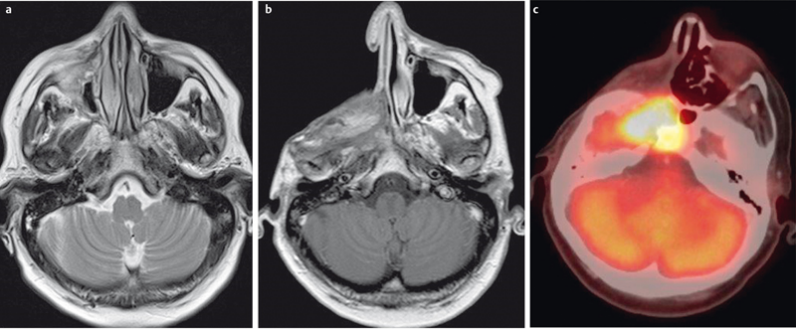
46-year-old female patient with chondrosarcoma of the right maxillary sinus. a) Preoperative axial T2w image demonstrates an inhomogeneous tumor with discrete infiltration of the surrounding soft tissue. b) Contrast-enhanced T1w follow-up image demonstrating extensive scar formation which cannot be differentiated from recurrent tumor. c) FDG-PET/CT performed the next day demonstrating increased metabolism within the scar formation, indicating recurrent disease, which was confirmed by surgery.
